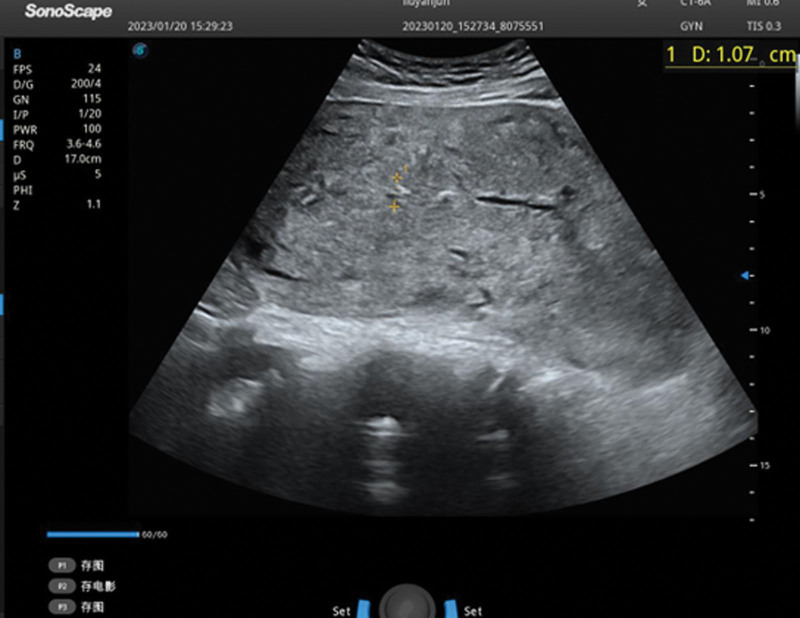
Three days postpartum, no obvious abnormality of the uterus or adnexa confirmed by ultrasound examination.
